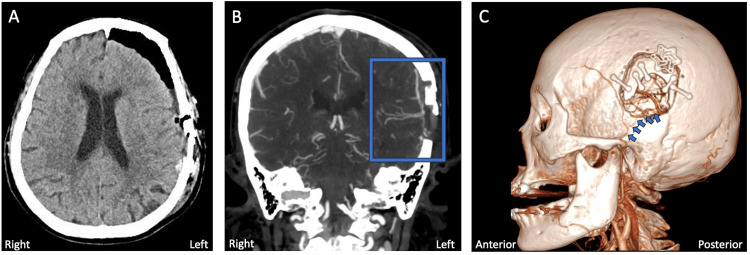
CT head imaging after STA-MCA bypass surgery A) Axial CT non-contrast imaging depicting left hemisphere post-surgical changes. B) Coronal CT angiography depicting anastomosis of the STA with the distal MCA. C) 3D rendering of sagittal CT angiography with patent STA-MCA anastomosis. The blue box focuses on the site of anastomosis; blue arrows track the cranial progression of the superficial temporal artery into the skull CT: computed tomography; STA: superficial temporal artery; MCA: middle cerebral artery
